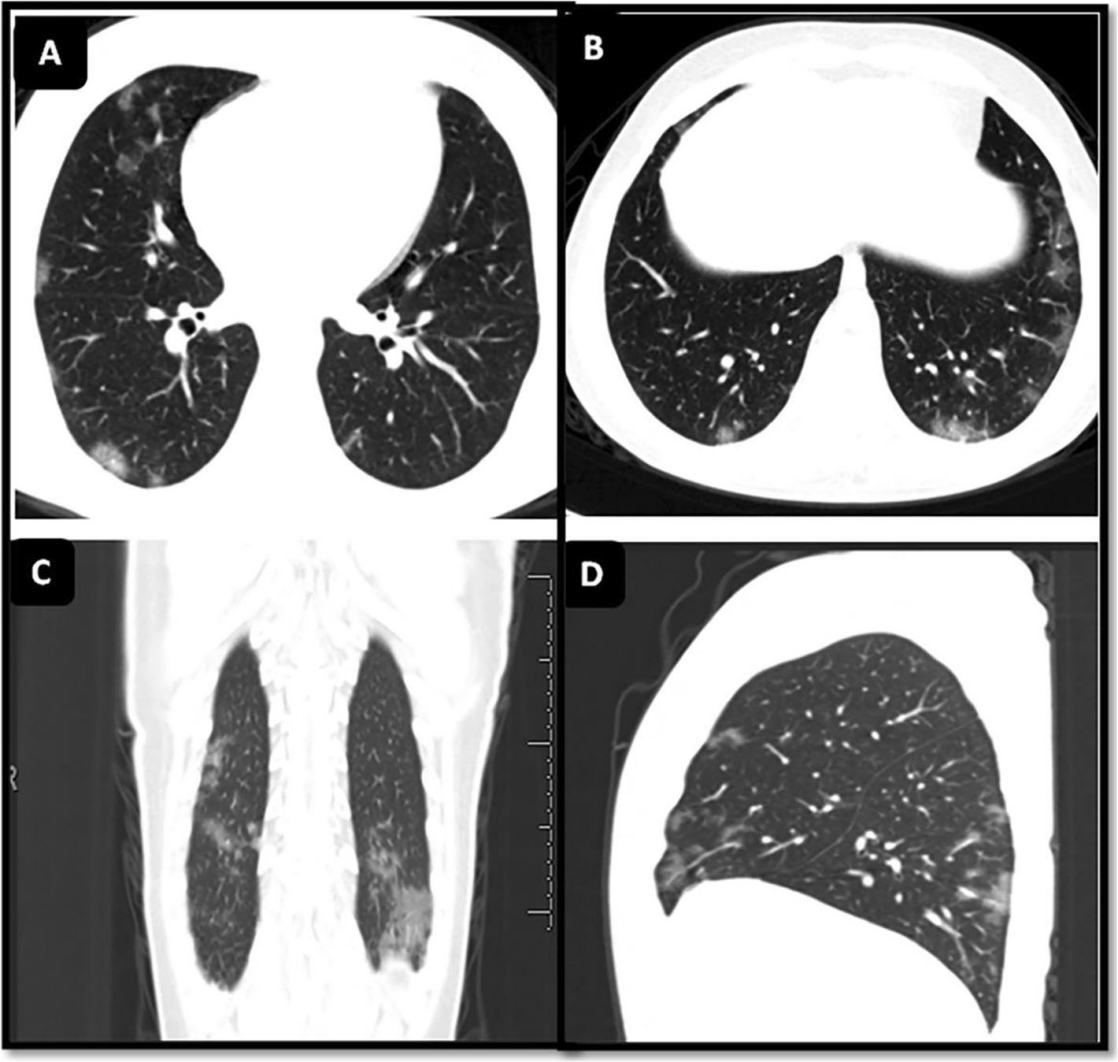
A male patient aged 8 years old with AML and under chemotherapy developed a COVID-19 infection and presented with fever and cough. Chest CT revealed multiple bilateral peripherally located ground-glass opacities; chest CT severity score was 5
